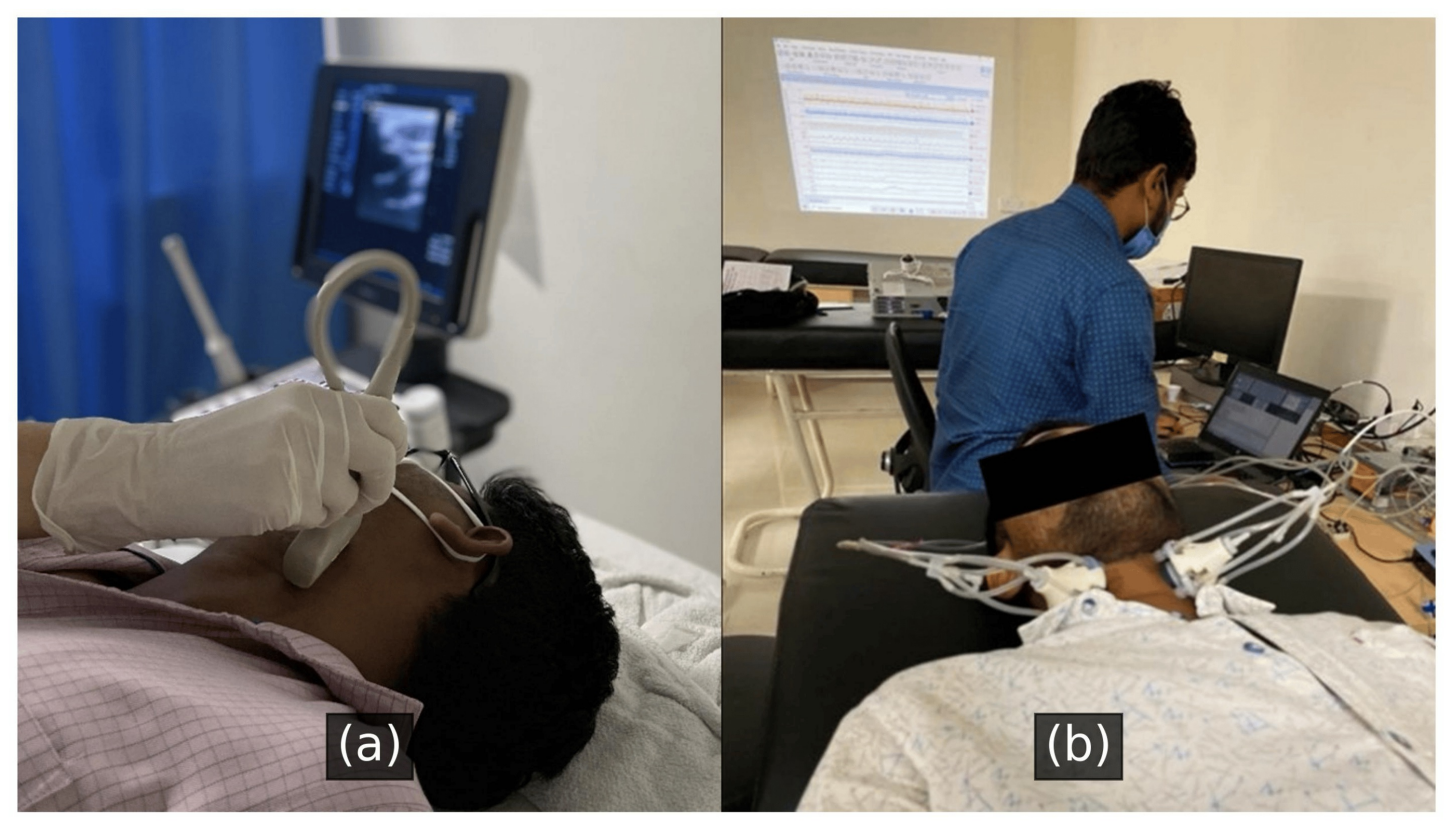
The device arrangement on test volunteers, along with the recording mechanism used in the study (a) Ultrasound-guided localization of the carotid baroreceptor prior to stimulation. A radiologist performs neck ultrasonography to identify the precise location for optimal placement of the stimulation device. (b) Bilateral carotid baroreceptor stimulation using the paired neck chamber device. The volunteer undergoes controlled stimulation while physiological signals, including heart rate and blood pressure, are continuously recorded for analysis.
